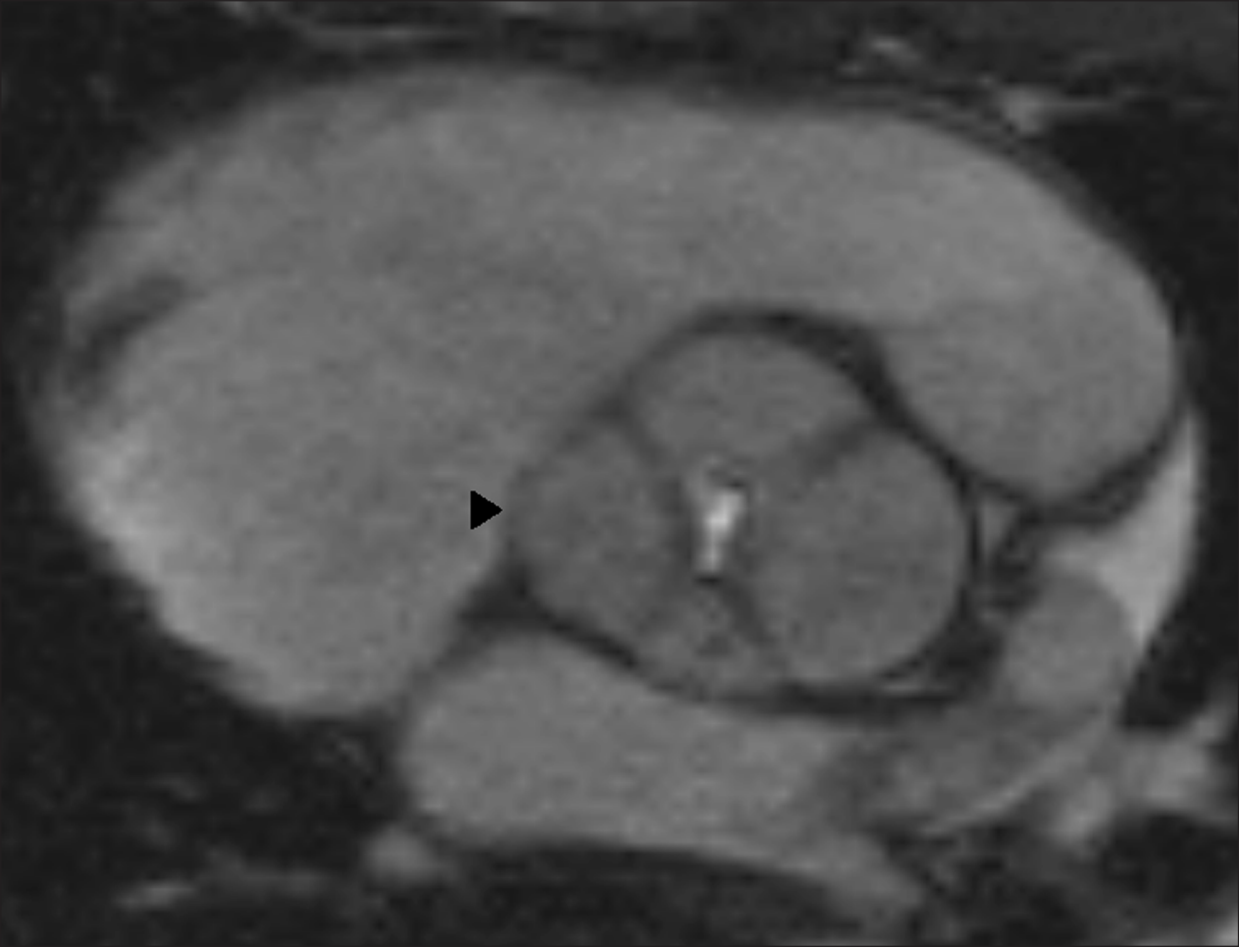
Cine MRI image in the aortic valve plane demonstrating the four-leaf-clover image (arrowhead) of the quadricuspid valve in diastolic phase. It is considered a type D QAV because there is one large cusp, two medium-sized cusps, and one smaller cusp.
